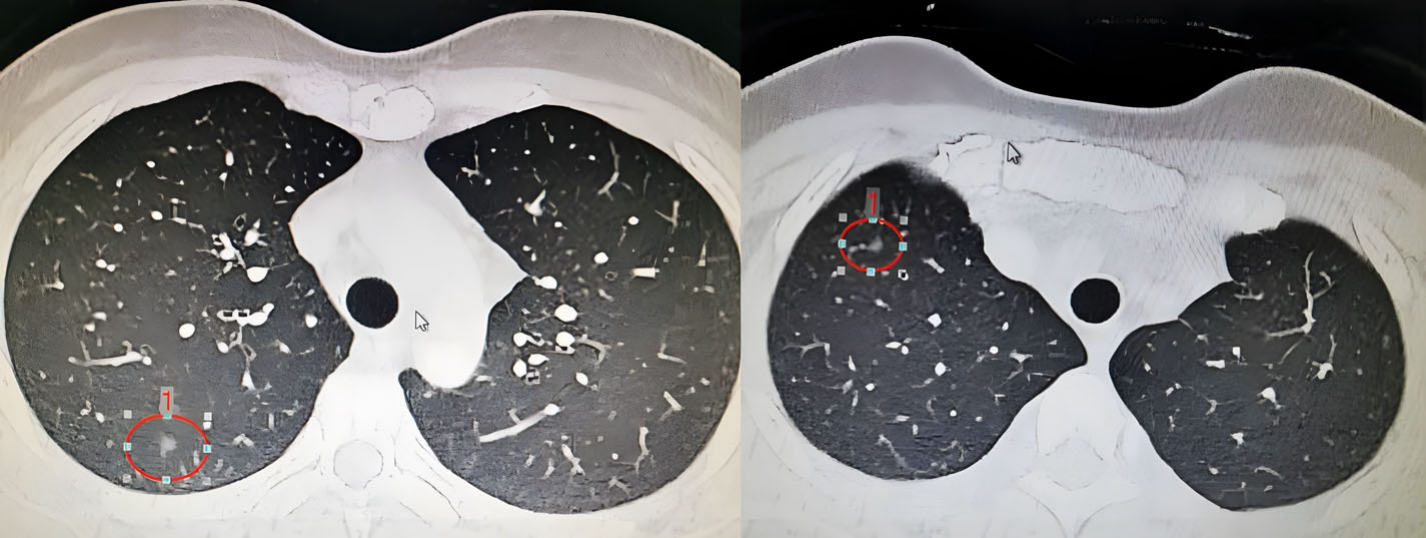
(a) Preoperative lung CT scan for pulmonary node in RS2a; (b) Preoperative lung CT scan for pulmonary node in RS3b
